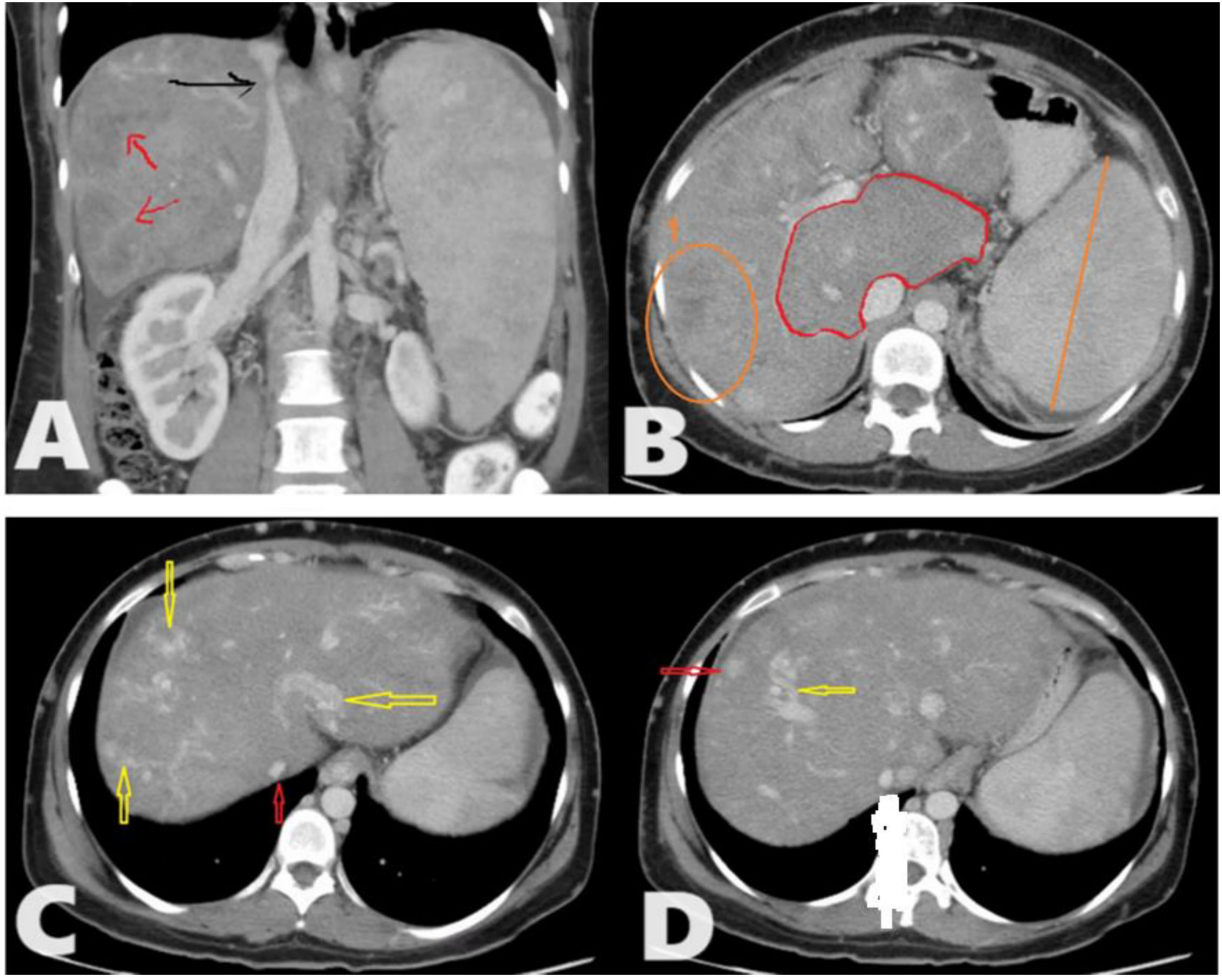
A total of 35 years female presented to the radiology department with chronic abdominal pain. Triphasic computed tomography (CT) abdomen reveals; (A) Chronic Budd-Chiari syndrome (BCS) manifested as Enlarged liver with heterogeneous enhancement and a patchy hepatogram with areas of hypoperfusion (Red arrows). The hepatic part of inferior vena cava (IVC) shows a focal stenosis which may indicate the presence of an IVC web (Black arrow). (B) The caudate lobe is disproportionately enlarged compared to the rest of the liver. No IVC/portal vein thrombosis. (C) There are multiple intrahepatic collateral vessels, some of which drain directly into the IVC (yellow arrows) and narrow IVC (red arrow) with no enhancement of the hepatic veins (HVs) (inability to identify HVs). (D) There are multiple enhancing nodules spread throughout the liver (Red arrow) as an example.
